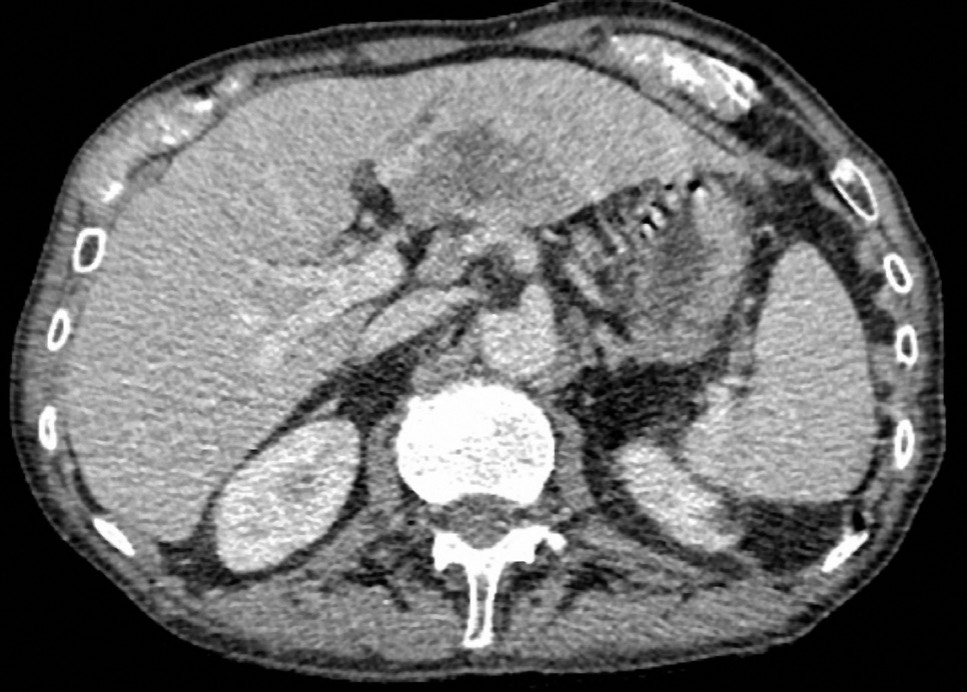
TAP CT scan - venous phase: hypodense elongated structures in the left hepatic lobe; adjacent to this area, there is a round-oval lesion (16 mm).
